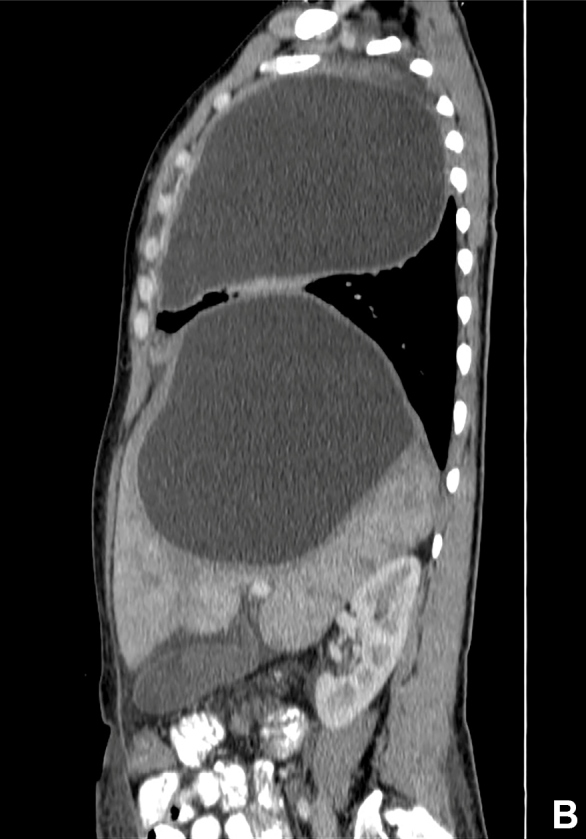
Computed tomographic scan of the chest showing one cyst (14 × 11 cm) in the right lobe of the lung.
